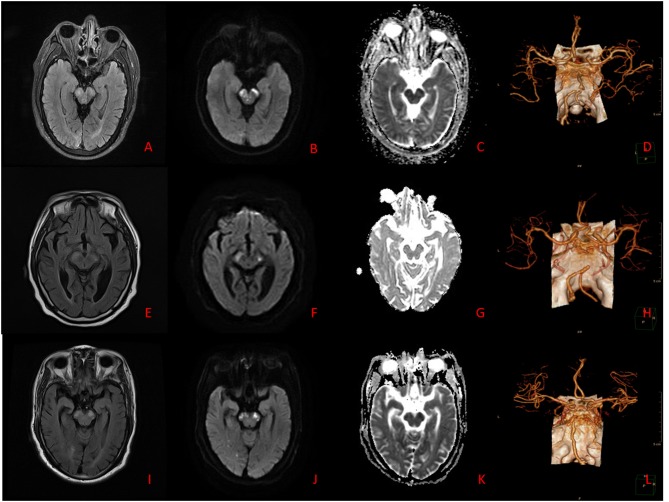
(A–D) Case 7. Brain MRI: The lesions appear hyperintense on T2WI, T2-FLAIR, and DWI, and hypointense on ADC. Brain CTA: Stenoses are located in bilateral VA. (E–H) Case 8. Brain MRI: The lesions appear hyperintense on T2WI, T2-FLAIR, and DWI, and hypointense on ADC. Brain CTA: Stenosis is located in BA. (I–L) Case 10. Brain MRI: The lesions appear hyperintense on T2WI, T2-FLAIR, and DWI, and hypointense on ADC. Brain CTA: Stenoses are located in right VA and BA.
